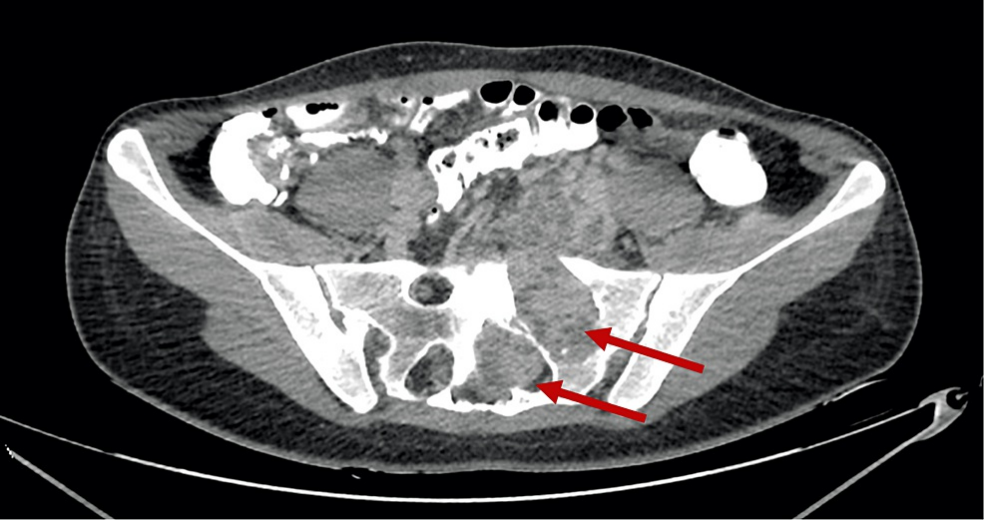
CT of the pelvis (axial view). The arrows indicate the intrasacral tumor obturating the spinal canal and sacral foramina. CT, computed tomography
